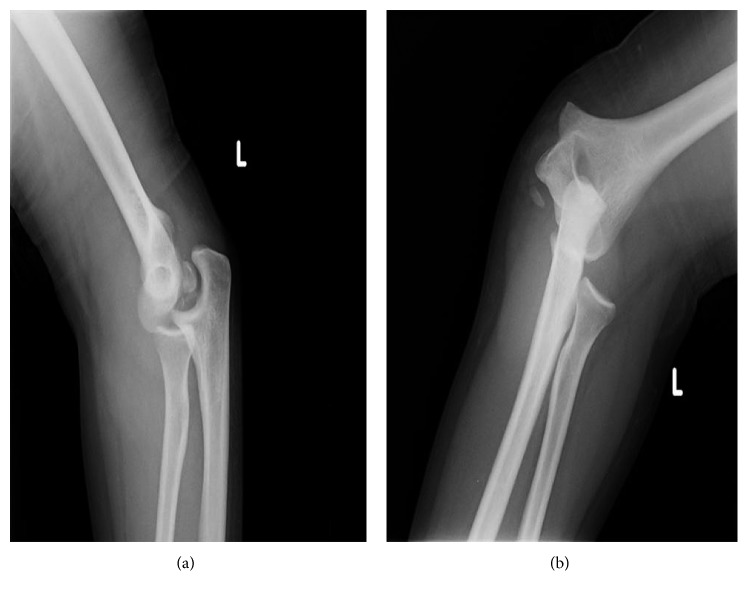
Lateral and anteroposterior radiographs of the left elbow joint showing posterolateral fracture dislocation with intra-articular entrapment of the avulsed medial epicondyle fragment. True lateral and anteroposterior radiographs could not be obtained due to the pain and the dislocation.
